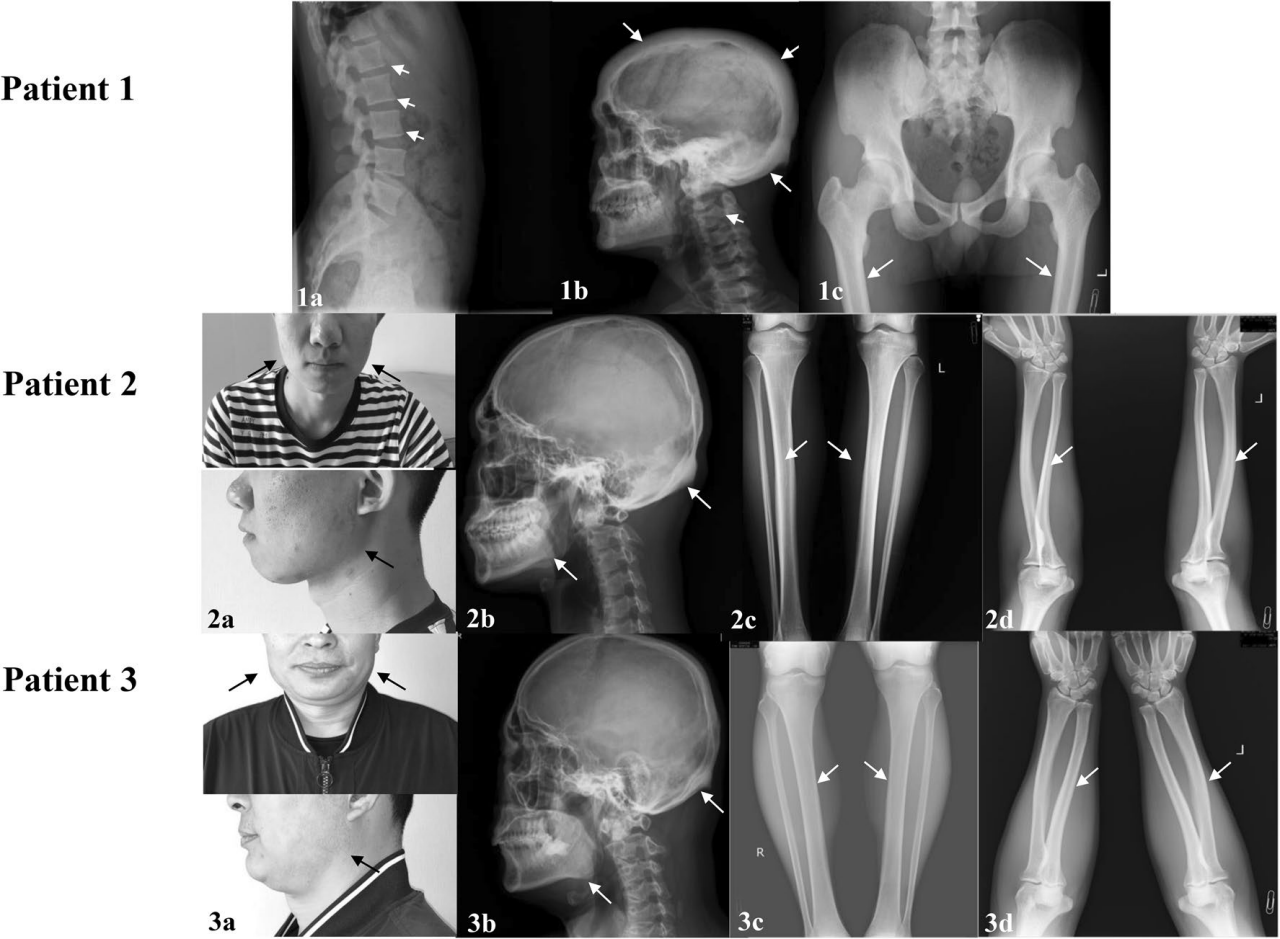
The physical findings and radiographic changes of patients in this study. (1a–c) The X ray films of lumber spine, cranial vault and hip of patient 1. The thickened bone cortex, narrowed bone marrow cavity of long bones. (2a、3a) The positive signs of patient 2 and 3. Mandibular enlargement and gonial angle protrusion. (2b–d) The X-ray films of long bones and skull of patient 2. A symmetrical cortical thickening of long bones, the skull with a normal external shape. An enlarged mandible could be seen in the X-ray of skull. (3b–d) The X-ray films of long bones and skull of patient 3. The thickening endosteum of the radius, ulna and the skull. A thickening bone cortex of the femur and fibula, and an enlargement of the mandible. The abnormal findings were indicated by *arrow*
